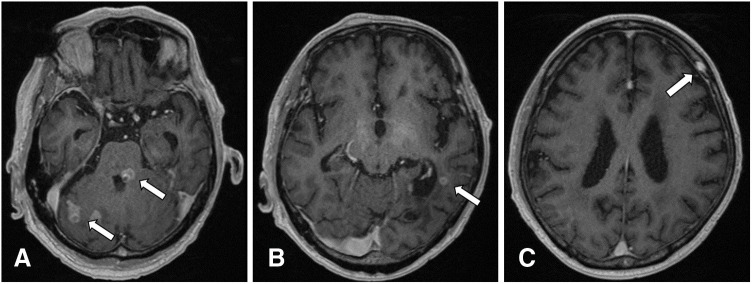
Head magnetic resonance imaging after secondary treatment. Multiple new lesions within the brain (arrows). (A) Right and left-brain ventricles. (B) Near left lateral ventricle. (C) Left frontal periosteum.
